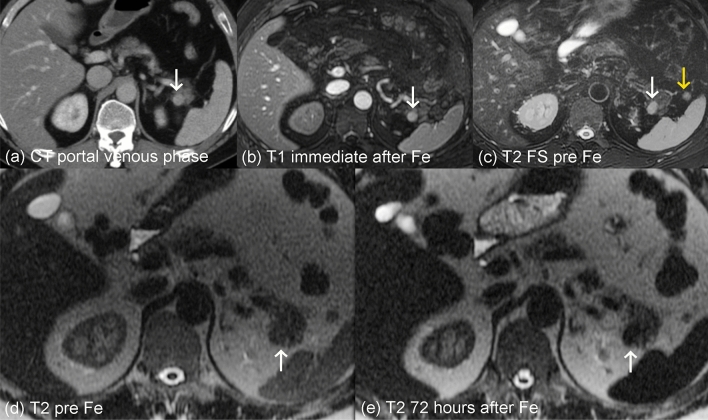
Typical imaging behavior of an intrapancreatic splenule on feMRI (pt. 2). CT shows enhancing lesion (white arrow, a), while T1w imaging shows strong enhancement immediately after administration of ferumoxytol (Fe) in an intravascular phase at MRI#1, similar to the spleen (b). On T2w imaging, the lesion is isointense before contrast (c, d) and demonstrates decreased signal, also similar to the spleen, 72 h after the administration of ferumoxytol (e). Note an additional splenule in the hilus of the spleen (yellow arrow)
